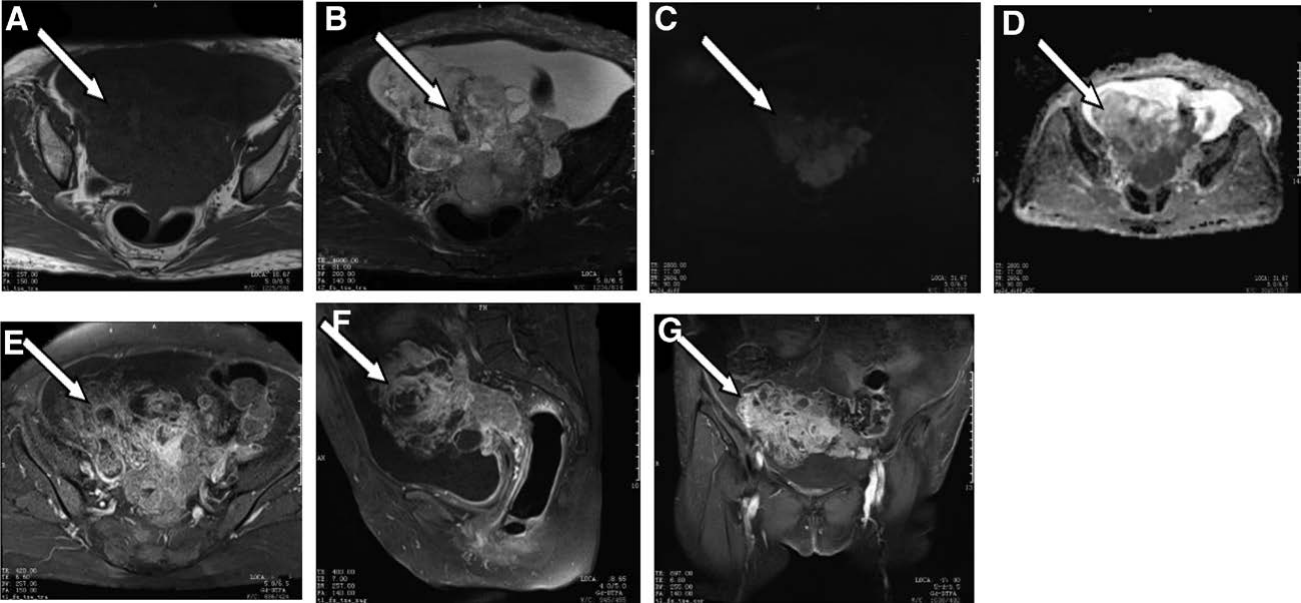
Case 1. Malignant mixed Mullerian tumor of the right adnexal region. (A) T1WI. (B) FS-T2WI. (C) DWI. (D) ADC. (E–G) Contrast enhancement. ADC = apparent diffusion coefficient, DWI = diffusion-weighted imaging, T1WI = T1-weighted image, T2WI = T2-weighted image.
